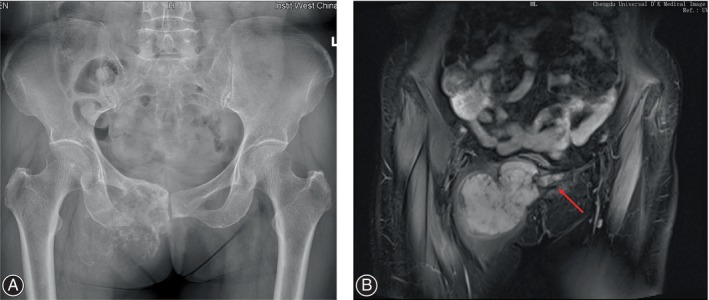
The X‐ray (A) and the MRI (B) demonstrated that the tumor invaded bilateral pubic (red arrow).
